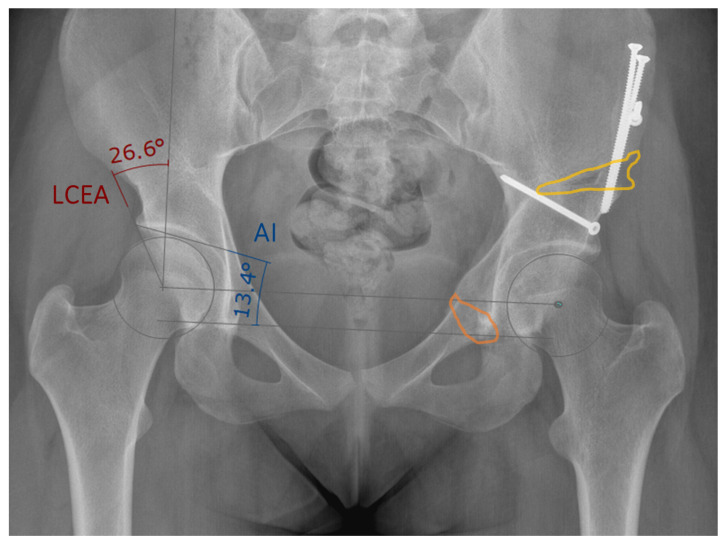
Example of a postoperative AP pelvic radiograph after PAO, showing the lateral center-edge angle (LCEA) and acetabular index (AI) measurement. On the left hip joint, the area where the supraacetabular oteotomy has been performed is shown in yellow. The area of the pubic osteotomy is marked in orange.
